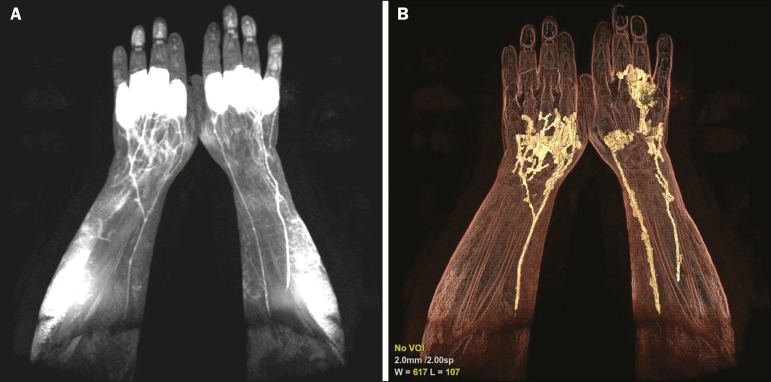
A 54-year-old man with rheumatoid arthritis. A: Maximum intensity projection of a volumetric T1-weighted sequence, with a slice thickness of 1 mm, showing normal lymphatic vessels in both upper limbs, without signs of obstruction. B: The same acquisition with three-dimensional enhancement techniques.
